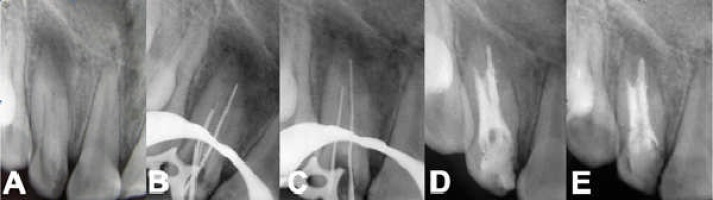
A) Preoperative radiograph of maxillary lateral incisor showing dens invaginatus with periapical radiolucency, B and C) K-files in both canals, D) Radiograph immediately after obturation of primary root canal, and E) one-year follow-up radiograph of maxillary lateral incisor. Radiograph reveals a reduction in size of the radiolucent area
